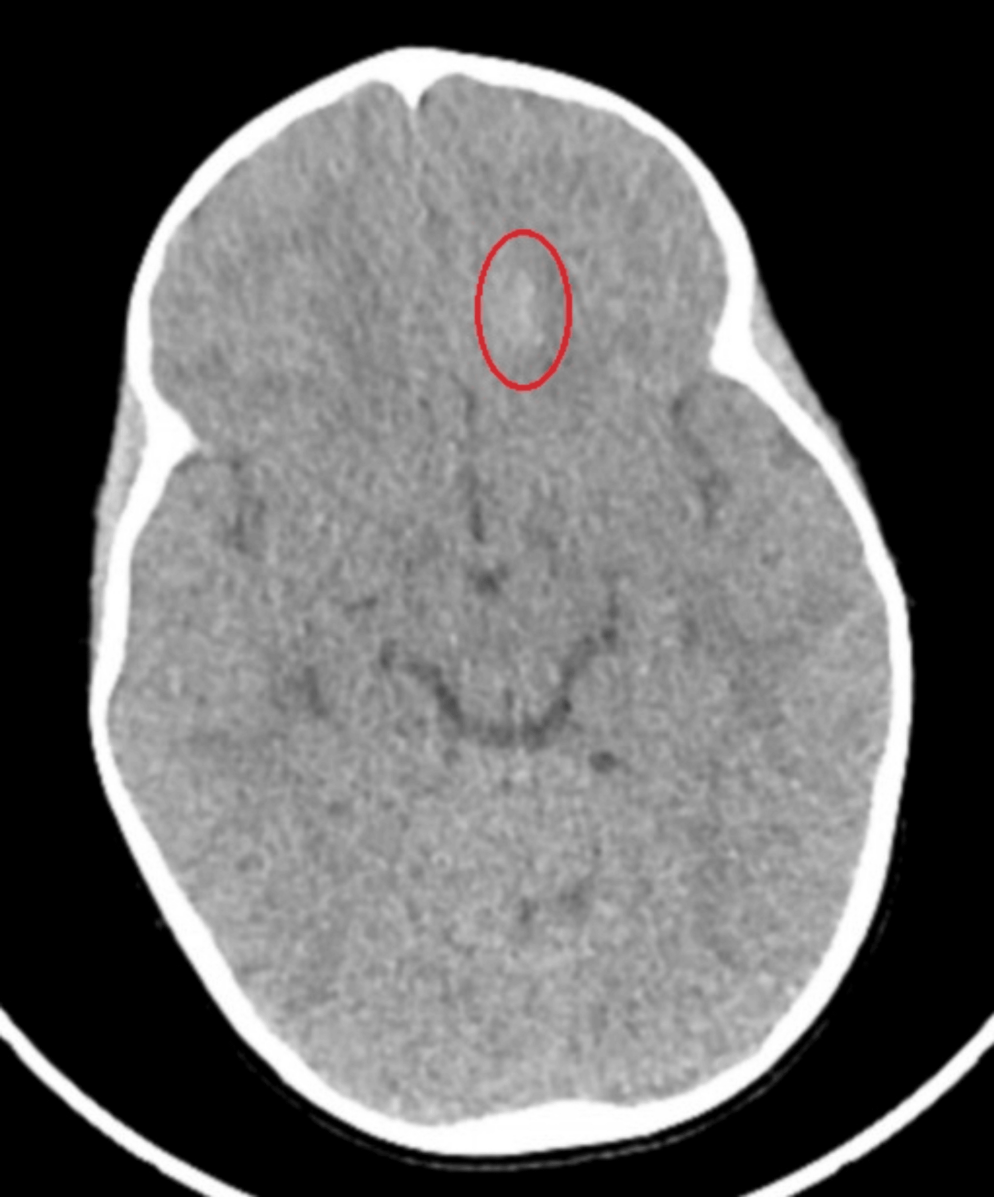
Non-contrast CT scan of the head: axial slice at the level of the third ventricle The left frontal lobe shows an ill-defined ovoid focus of subtle hyperdensity (red circle) without mass effect or surrounding edema. This finding is asymmetric compared to the right lobe, and no other abnormalities are detected in the rest of the brain parenchyma. This is a nonspecific finding. Differential considerations include artifacts, calcification from a remote insult or underlying mass, or, less likely, age-indeterminate blood products. An MRI would be warranted if there is a concern about bleeding.
